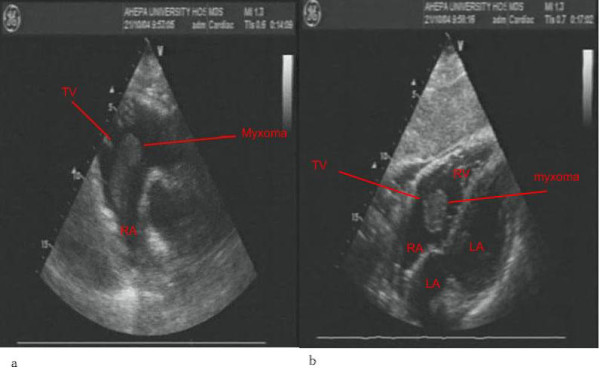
Myxoma in the right atrium. a. Transthoracic echocardiographic study, parasternal short axis view. Right atrial (RA) myxoma seen protruding across the tricuspid valve (TV) into the right ventricle. b. Transthoracic echocardiographic study, subcostal 4 chamber view. Right atrial (RA) myxoma seen protruding across the tricuspid valve (TV) into the right ventricle (RV) during diastolic period. LA refers to left atrium and LV to left ventricle.
